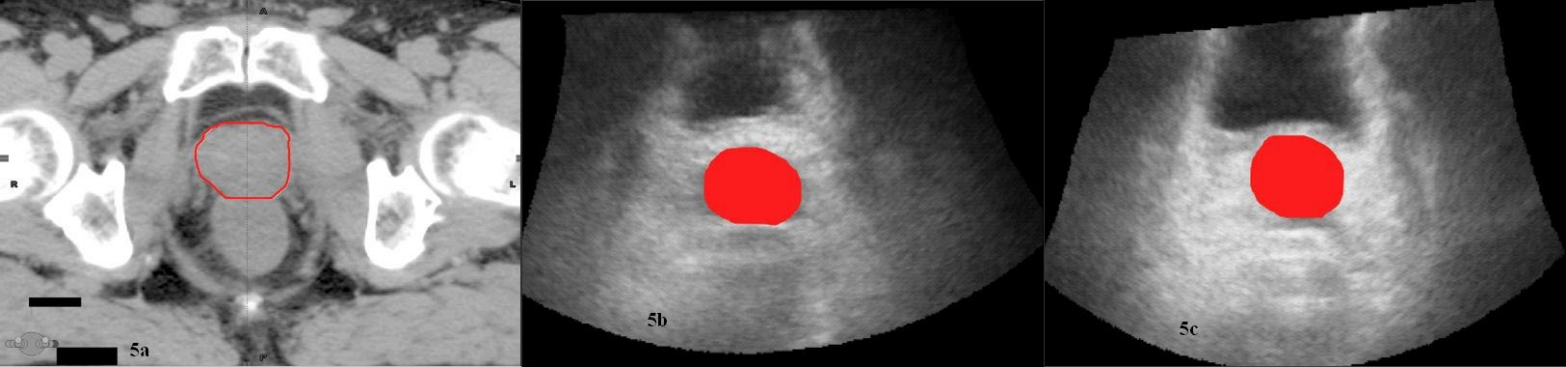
Representative images drawn from the RMr dataset showing the prostate localization provided by a tracking CT scan (a) and its two associated ultrasound tracking scans: US1 (b) and US2 (c).
